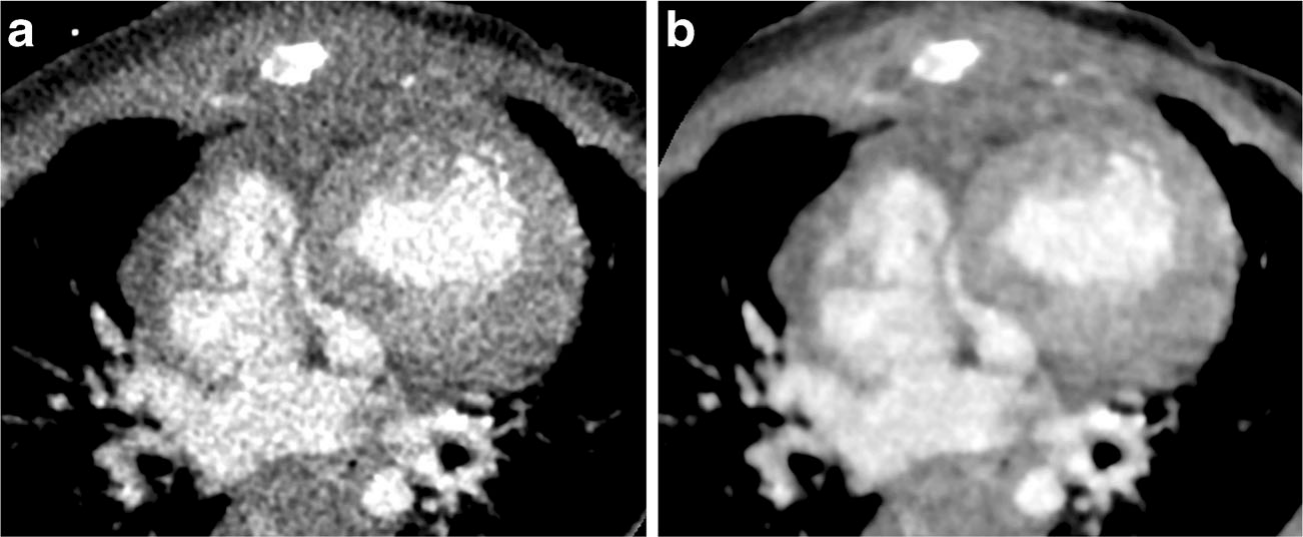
Axial contrast-enhanced CT images in a 4-month-old boy with hypoplastic left heart syndrome. a Hybrid iterative reconstruction. b Full iterative reconstruction. Noise, graininess, and contrast resolution between cardiac and coronary structures and the lumen are significantly improved with full iterative reconstruction as compared with hybrid iterative reconstruction. The CT window settings of both figures are identical
